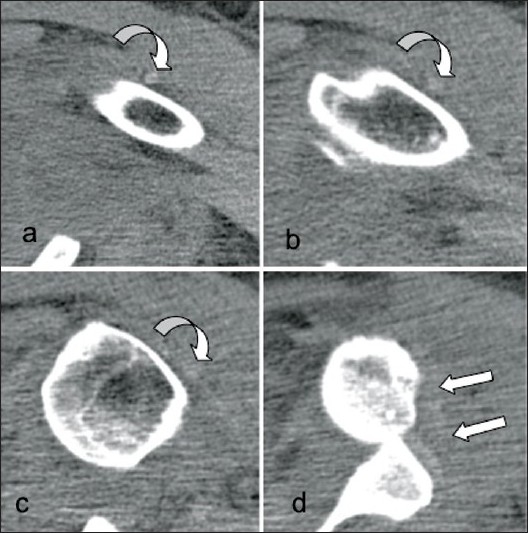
CT images obtained with axial 3 mm collimation demonstrate the long head of biceps tendon dislocated lateral to the (a) proximal humeral shaft and (b) bicipital groove as well as (c) posterior to the greater tuberosity and (d) Hill–Sachs defect. Note failed closed shoulder reduction with anterior subcoracoid dislocation of the humeral head
